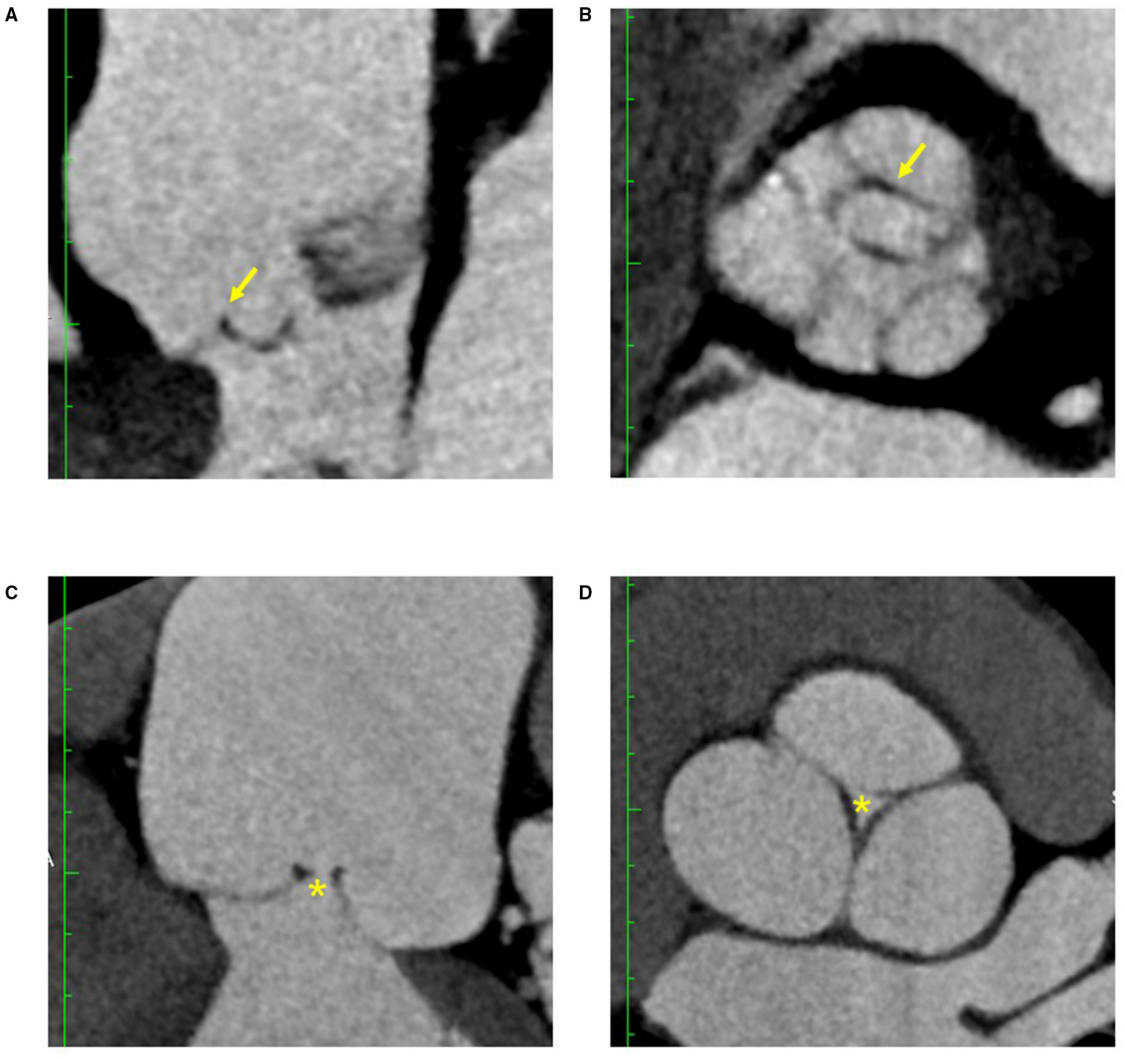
CT features of AR with eccentric jet and central jet. Bent leaflet belly indicated by arrows were both identified in long axis (A) and short axis (B) of reconstructed CT images in AR with eccentric jet. Loss of coaptation indicated by asterisks were both identified in long axis (C) and short axis (D) of the reconstructed CT images in AR with central jet. AR, aortic regurgitation.
